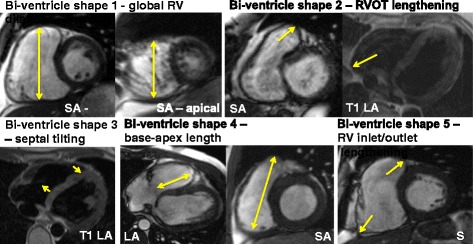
CMR of the patients with the largest shape loadings for each shape. The short axis (SA) views around the basal and apical regions showed global dilation in patient 26, the SA and T1-weighted long axis (LA) images for patient 24 displayed the elongation at the RV outflow tract (RVOT), the T1-weighted LA image of patient 3 showed the tilting of the RV from the apex to the base at the septum, the LA view showed shortening of the RV (shown in the SA view) for patient 4, and the SA view of patient 16 showed dilation at the RV inlet and outlet. Note that the T1 images shown here are for visualisation purposes only, but were not used as a part of this study
